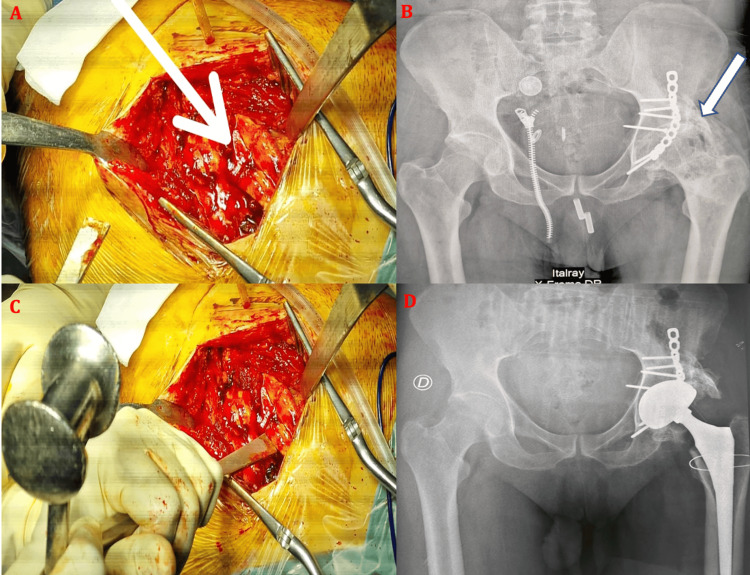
(A) Intraoperative view through a posterolateral Moore approach showing the presence of a bony bridge extending from the greater trochanter to the acetabulum (white arrow), corresponding to heterotopic ossification as demonstrated on the anteroposterior pelvic radiograph (B), in a 38-year-old male patient. Careful release was performed using an osteotome (C) to allow adequate exposure of the pathological hip and subsequent implantation of the total hip arthroplasty (D).
